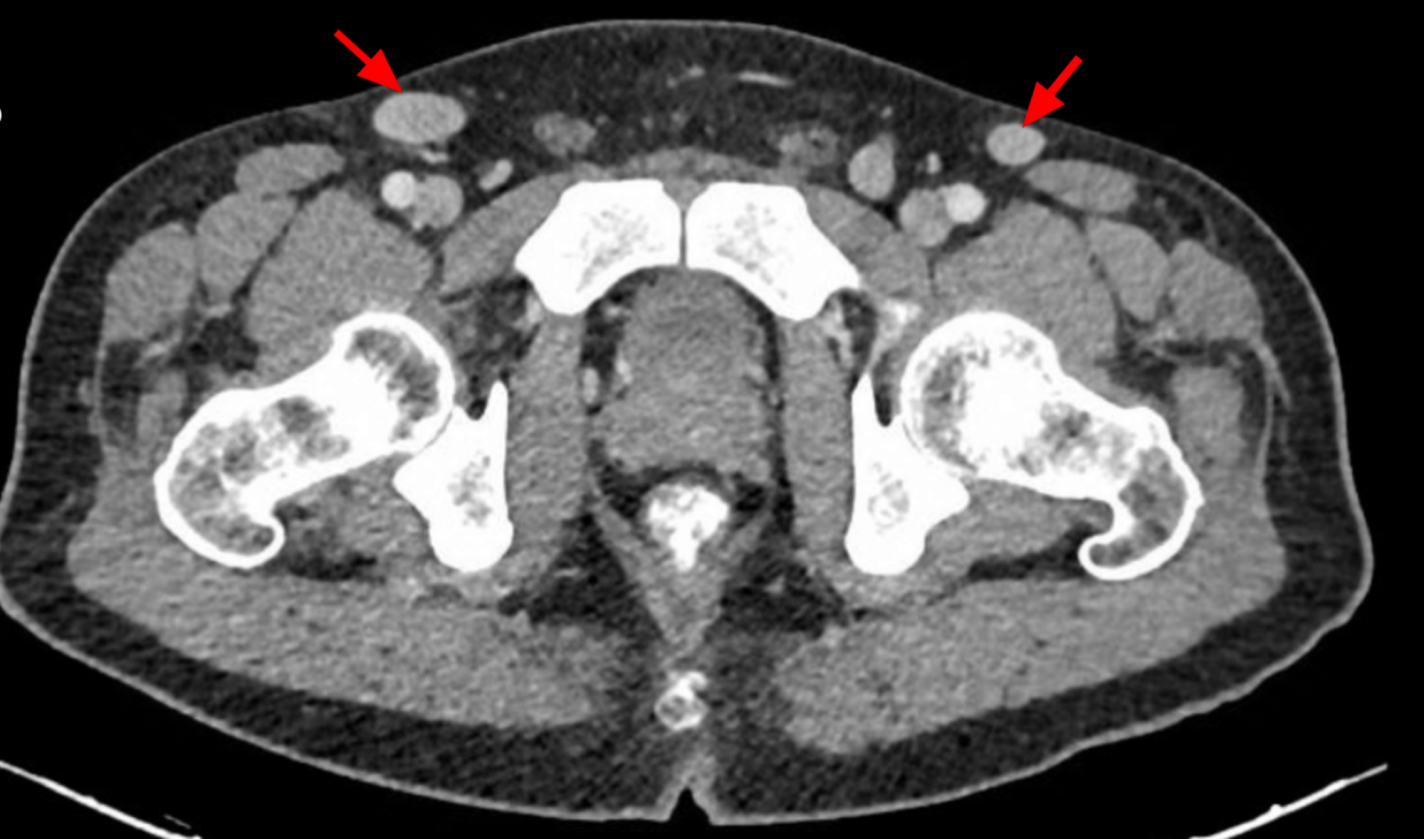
Computed tomography (CT) scan of the pelvis Red arrows: inguinal lymph nodes.
